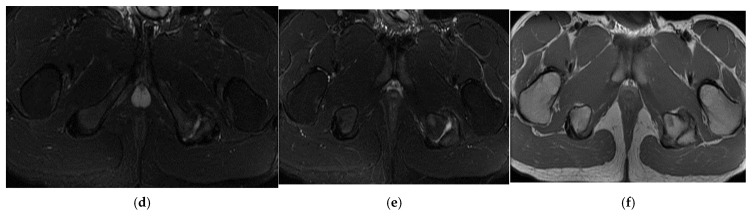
(a) Pelvic MRI: coronal stir acquisition, (b) pelvic MRI: sagittal PD fat sat acquisition, (c) pelvic MRI: sagittal PD fat sat acquisition, (d) axial stir acquisition, (e) axial stir acquisition, (f) axial T1 acquisition.
